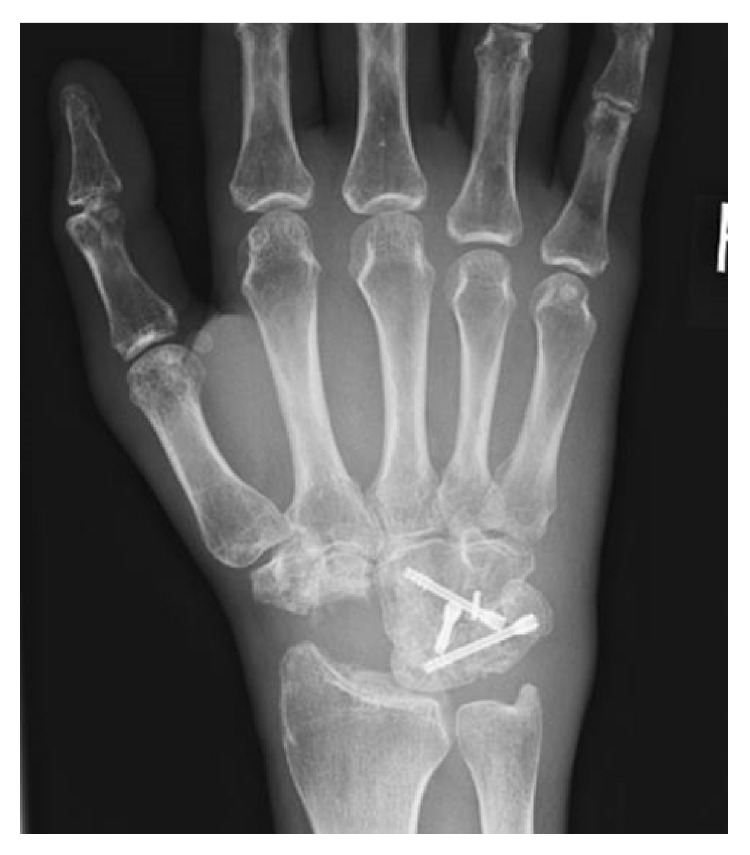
X-ray right wrist a.p. showing consolidated 4-corner fusion.
